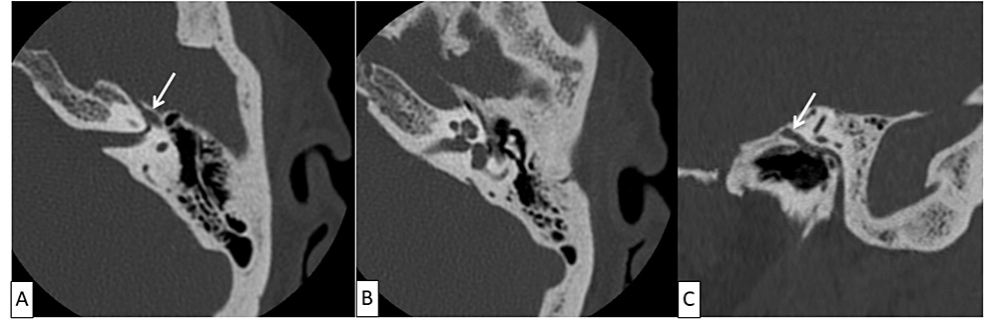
CT scan of the left petrous bone in axial sections: absence of anomalies in the left facial canal first portion and the geniculate ganglion (A), middle ear (B), and petrous bone and the second portion of the facial canal (C).
